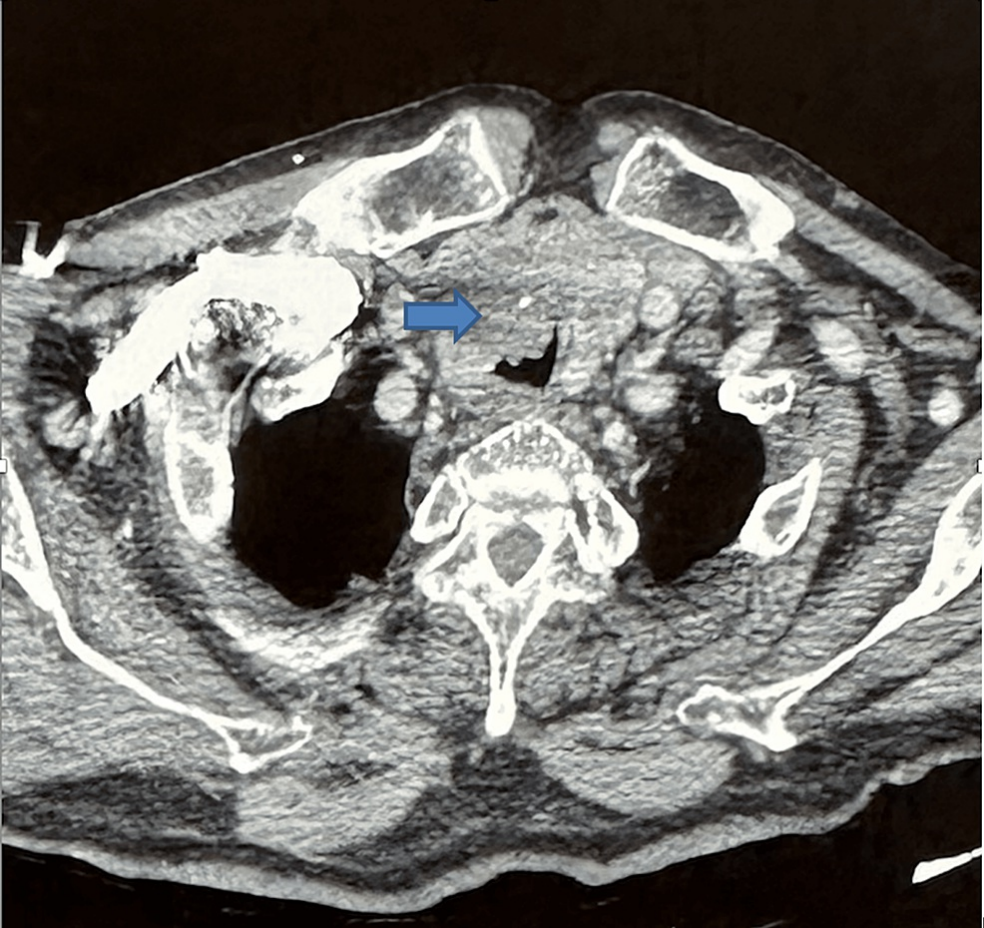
The CT scan revealed heterodense tissue lesion budding into the trachea at the level of D2, reducing the tracheal lumen and seeming to continue with the right isthmo-lobar thyroid parenchyma(blue arrow).
